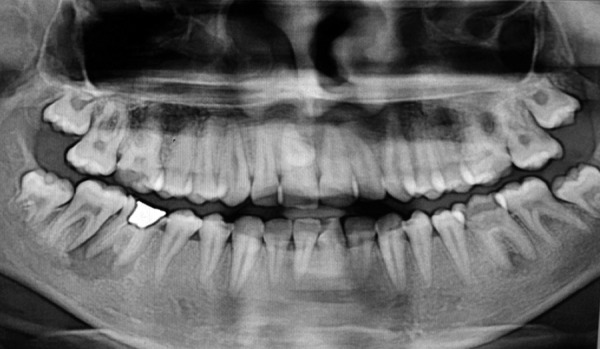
The effect or coronal restoration and root canal filling can be further evaluated with the OPGs shown here. Due to inadequate root canal fillings and improper CR, a periapical lesion more than 3 mm is seen in relation to mandibular right molar
